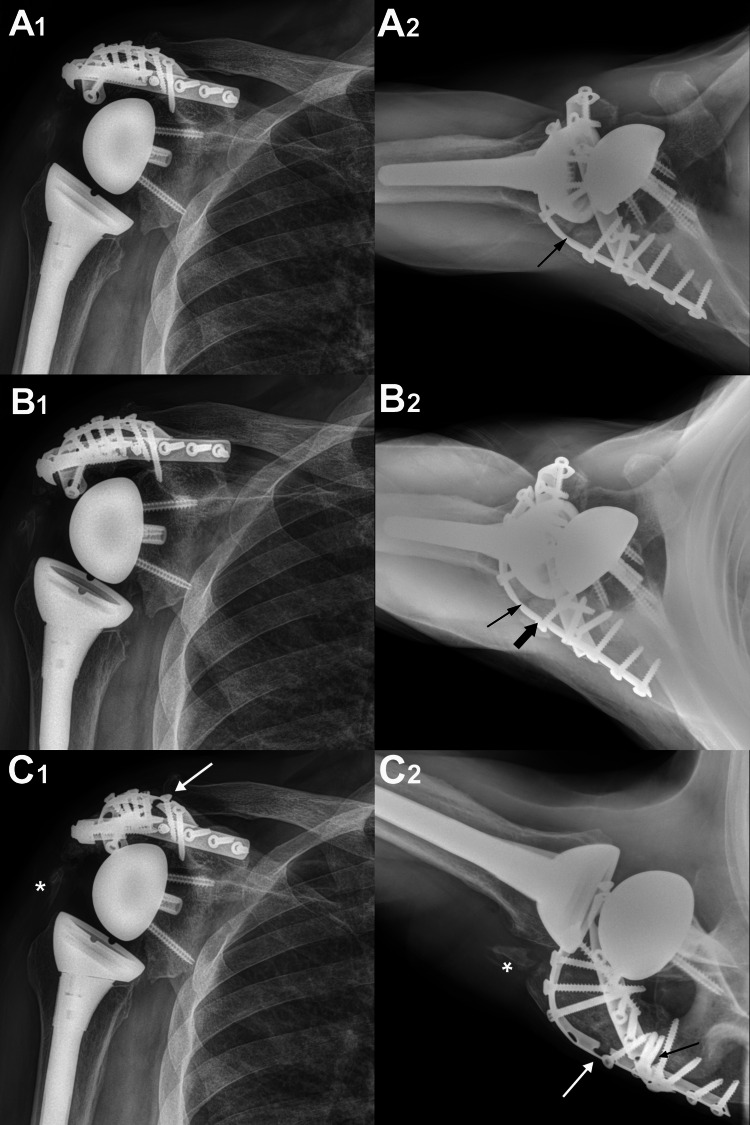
Our patient’s RTSA with double plating of the acromion fracture. Our patient’s RTSA with double plating of the acromion fracture in anterior-posterior and axillary lateral views at four months after the fracture reconstruction (A1 and A2), and at 11 months after fracture reconstruction (B1 and B2). The thin arrows shown in the axillary lateral views of A2 and B2 point to the grafted nonunion site. The thicker shorter arrow in B2 points to the first evidence of plate breakage.  Progressive calcification had occurred over this time interval (4-18-month follow-up period). The 18-month post-ORIF radiographs in part C show that fatigue fractures of the plates are now obvious (one arrow in C1; two arrows in C2), which are presumably from cyclic loading during his activities of daily living as a rancher. The nonunion subsequently consolidated (C2, beneath the plate hole without a screw). There was also mature-appearing heterotopic ossification in the upper-lateral deltoid (asterisks). RTSA, reverse total shoulder arthroplasty
